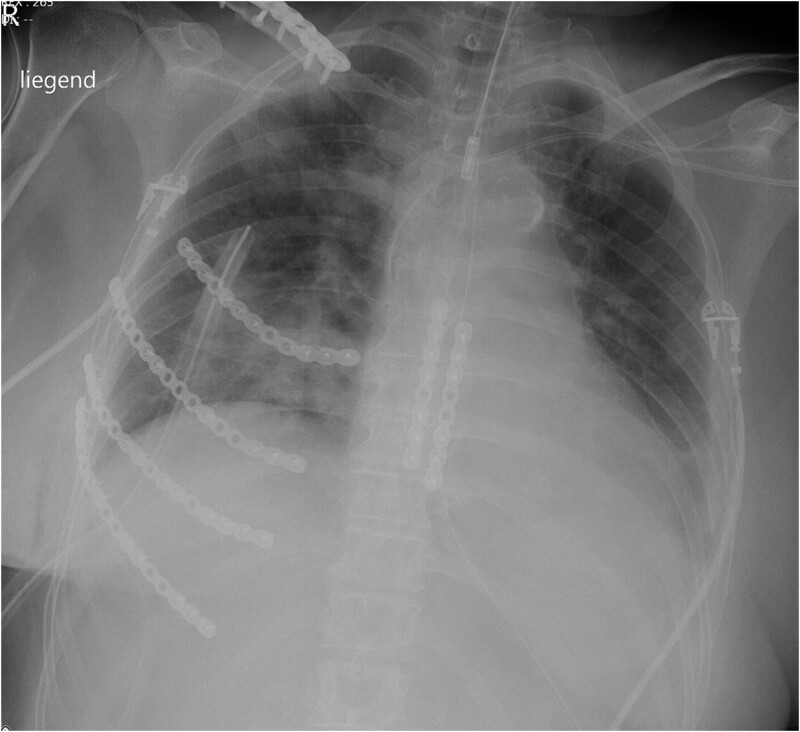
Postoperative X-ray after sternal stabilization, stabilization of ribs 3–6 on the right side and right clavicle.
